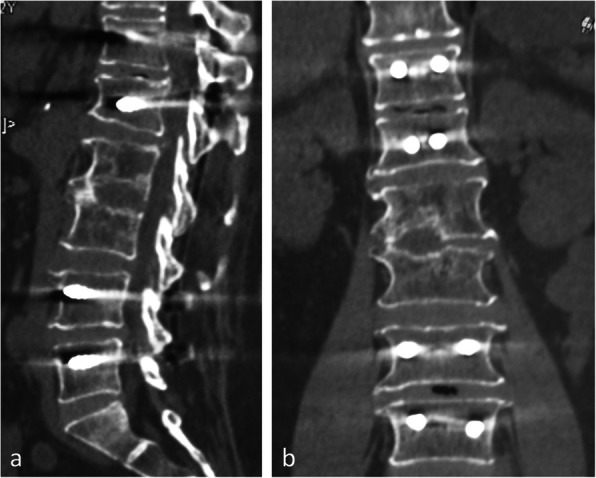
A 62-year-old female, sagittal (a) and coronal (b) CT reconstruction 1 year after surgery, showed spontaneous peripheral interbody fusion
